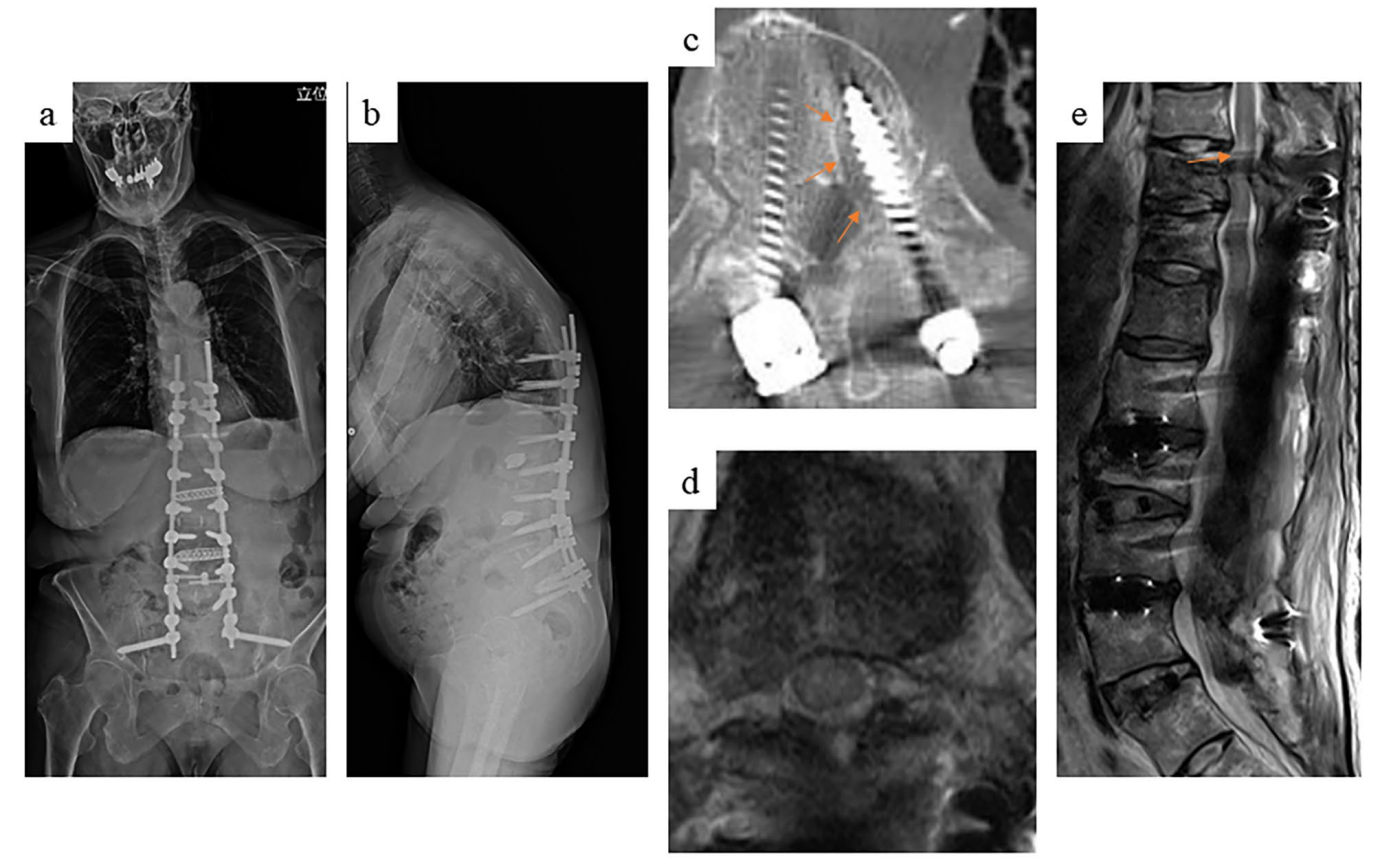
Standing X-ray 1 week after surgery showing back-out of the pedicle screws at the upper instrumented vertebrae (a, b). Computed tomography showing a clear zone around the loosened pedicle screws but no migration into the spinal canal (c). Magnetic resonance imaging also showed that the pedicle screws did not compress on the spinal cord in the axial view (d). However, several signal changes in the spinal cord were visible in the sagittal view (e)
